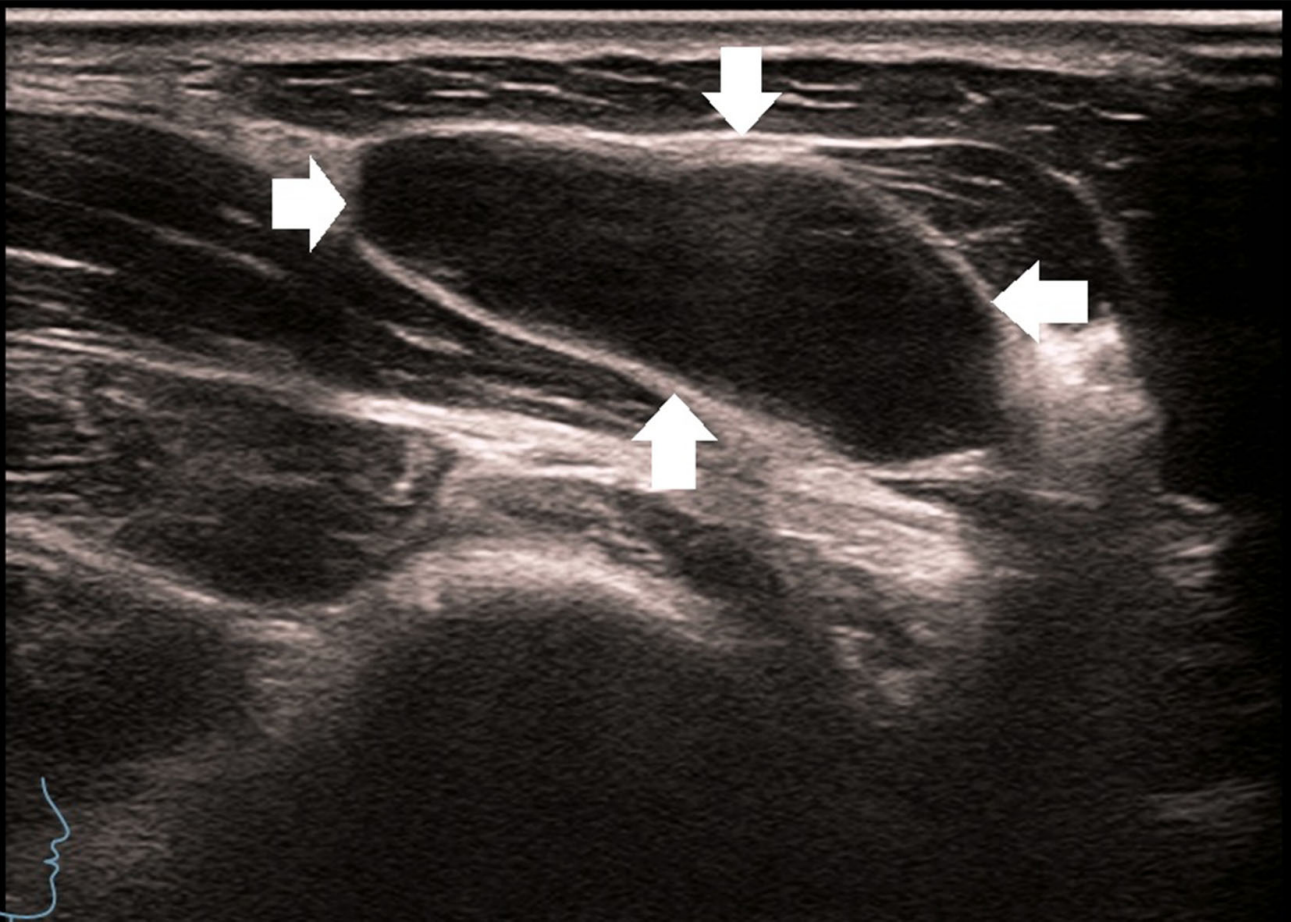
A 27-year-old man with enlarged lymph nodes in his right neck. Ultrasound showed significant enlargement of the lymph nodes, thickening of the cortex, disappearance of the lymphatic portal, and enlarged lymph nodes, as shown by the arrow.
